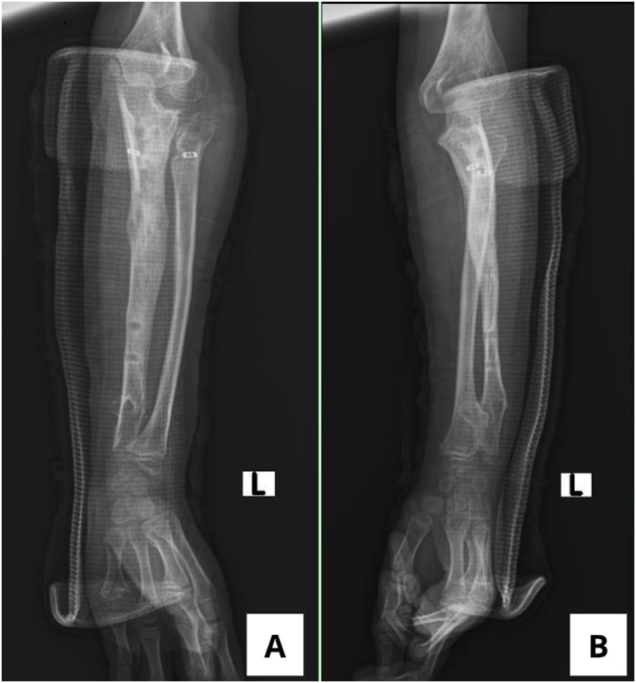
Post-secondary operative X-ray of the left forearm. (A) AP view and (B) lateral view show bone formation of the ulnar, and the fixation button was confirmed to be in the correct position.
